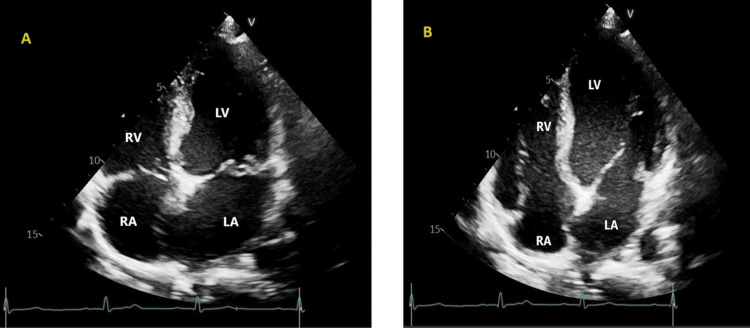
Follow-up transthoracic echocardiogram images (A) Systolic phase, when the heart muscle contracts; (B) Diastolic phase, when the heart muscle relaxes. LV: Left Ventricle; LA: Left Atrium; RV: Right Ventricle; RA: Right Atrium
